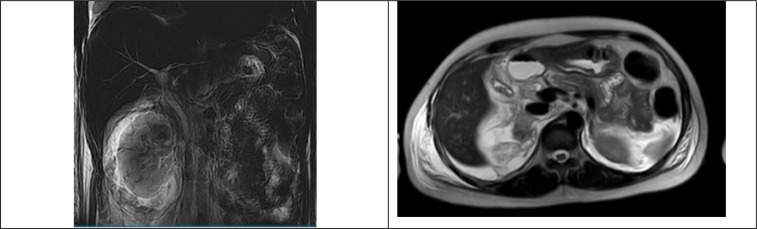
Coronal (left) and axial (right) views of abdominal Magnetic resonance imaging (MRI) at the time of admission
